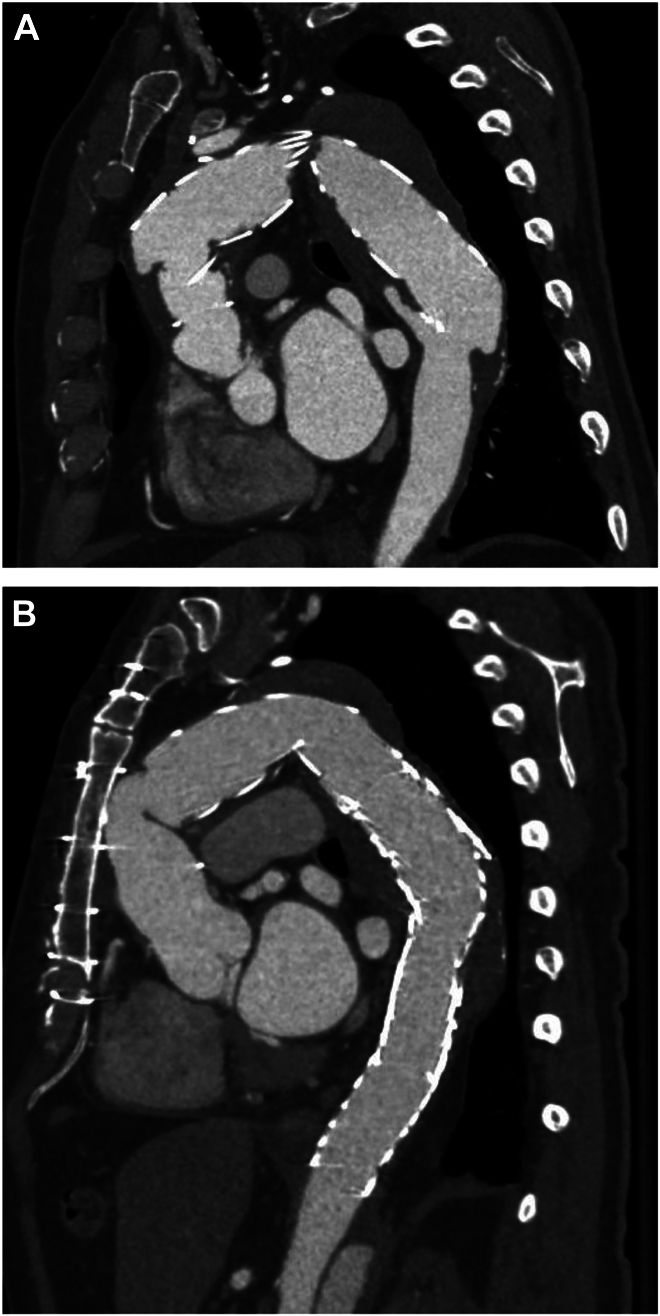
Computed tomography of hybrid arch repair. (A) Interval imaging 10 years after index hybrid arch repair surgery demonstrating proximal blowout and distal stent-induced aortic wall injury. (B) Imaging after definitive ascending aortic repairs.
